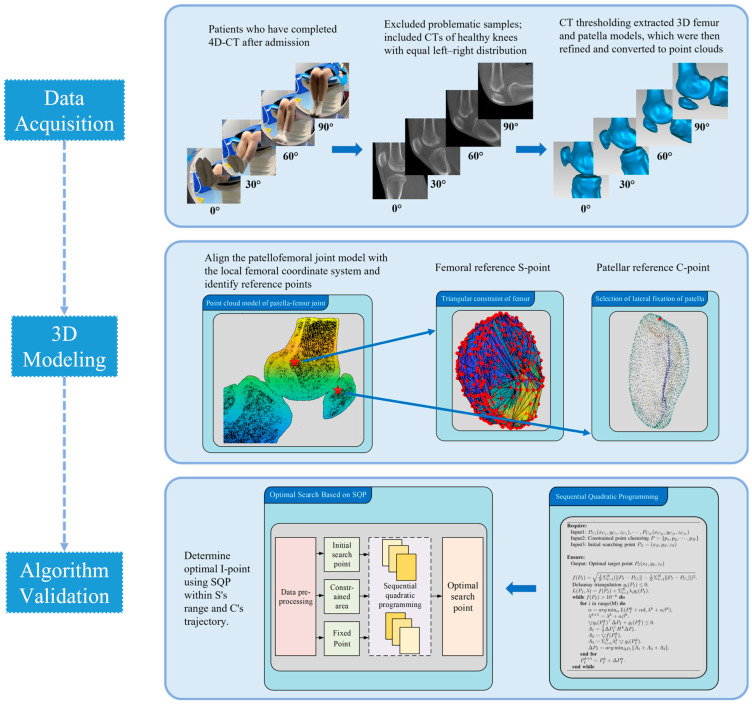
Experimental and computational procedures for 3D knee joint modeling. A total of 1382 knee CT models were studied for computation and analysis. 4D-CT was used to capture the patient’s active knee flexion from approximately 0° to 90°, and repair refinement was performed from the identified rough fuzzy 3D model. 3D models were converted to point clouds, identifying C-point (patella) and S-point (femoral reference). The individualized femoral I-point was calculated using a constrained SQP-based optimization method.
